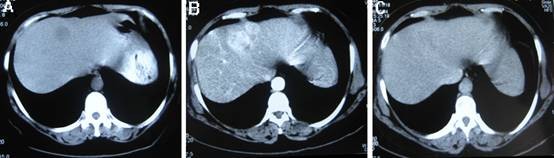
Computed tomography (CT) of the liver. A. Noncontrast CT image shows a low density mass in segment 4 of liver. B, C. Dynamic CT scans show enhanced of the nodule in the arterial phase and early washout in the portal phase.
